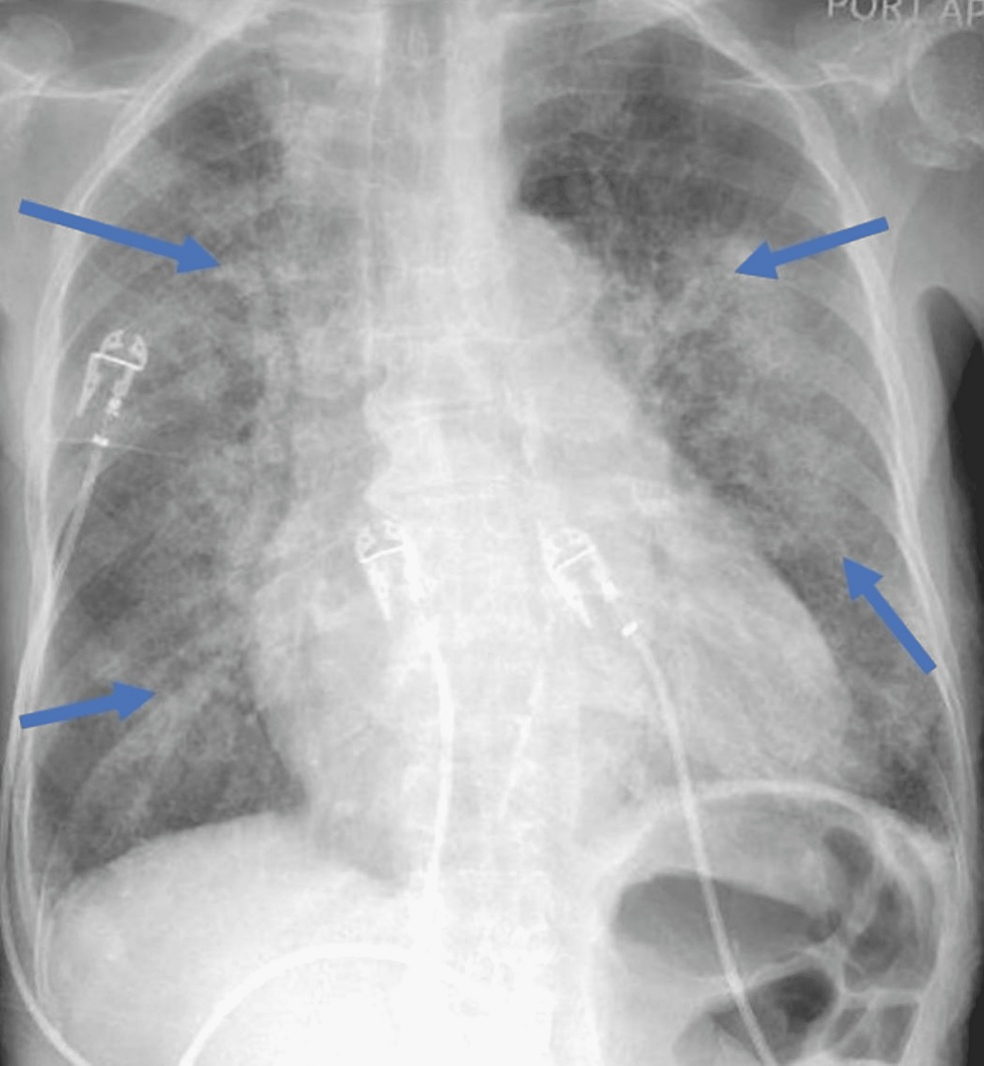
Chest X-ray showing bilateral patchy infiltrates and ground-glass opacities
